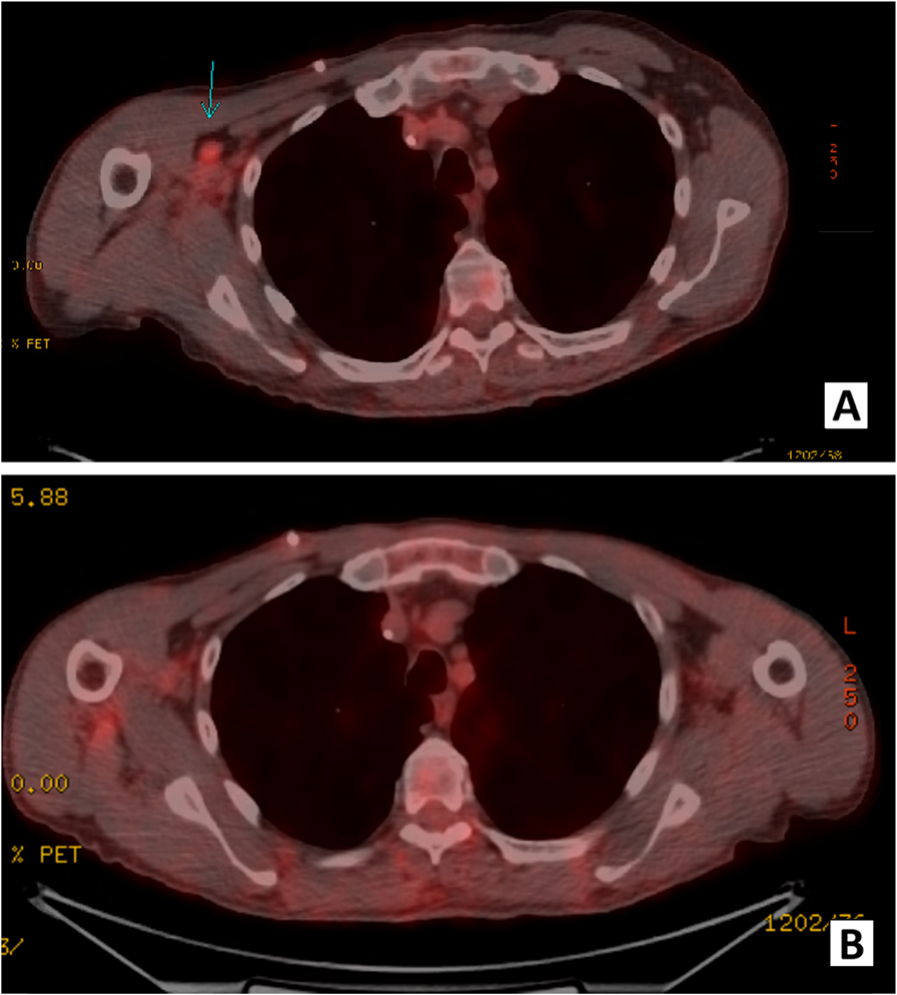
Post-metastasectomy surveillance PET scan demonstrating FDG avid right axillary lymph node (a) and resolution of FDG avidity as well as decrease in size of this node (b) following immunotherapy, indicating response to immunotherapy. This response persisted on subsequent PET scans and the patient remains disease free at 1 year after diagnosis of metastatic disease
